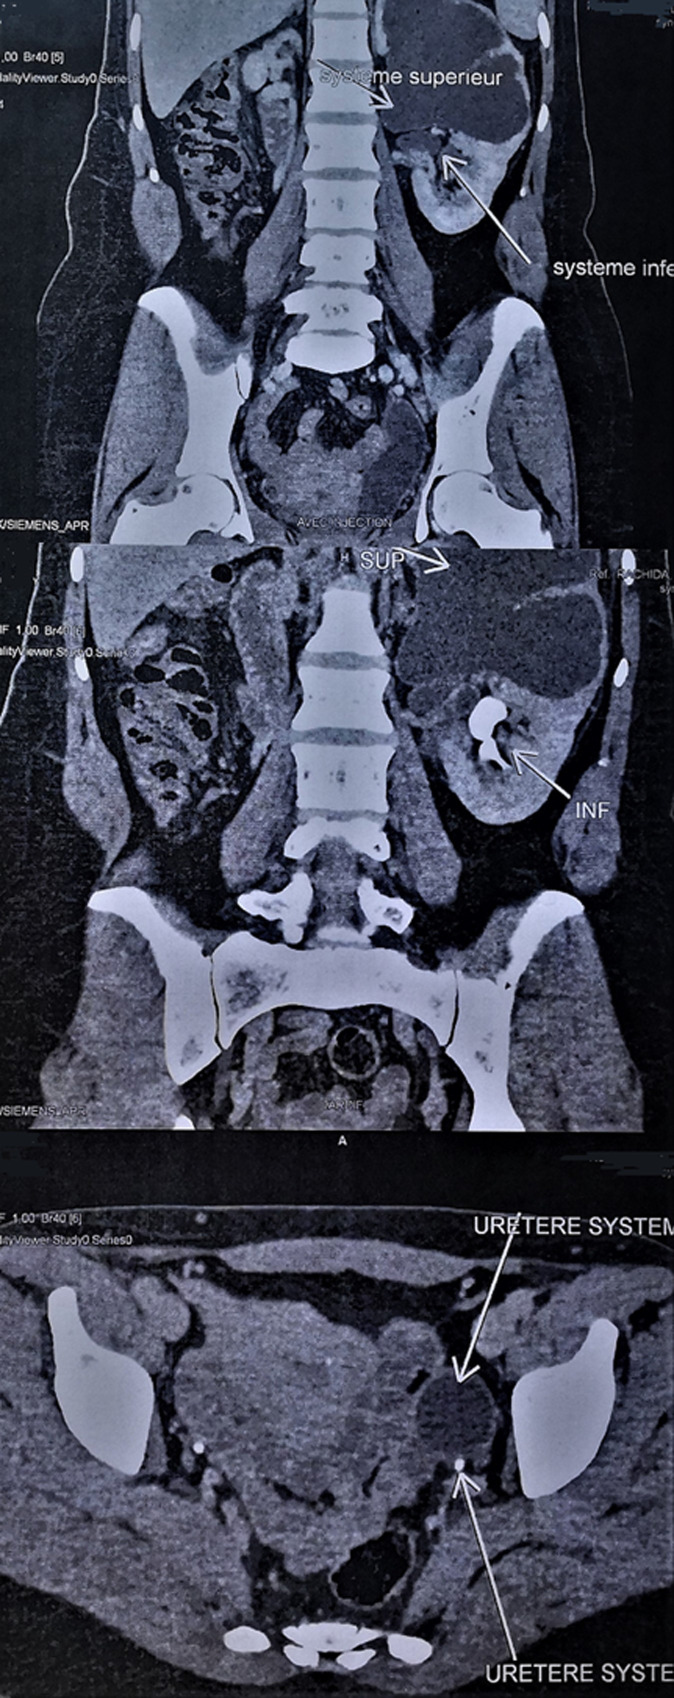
uroscan image of ureterocele and double renal system; computed tomography uroscan showed an enlarged left kidney measuring 169.5 mm in height and a double system with an ureterocele and ureterohydronephrosis of the upper system with a renal pelvis measuring 41.8 mm in anteroposterior diameter, a laminated aspect of the renal parenchyma and excretory delay
